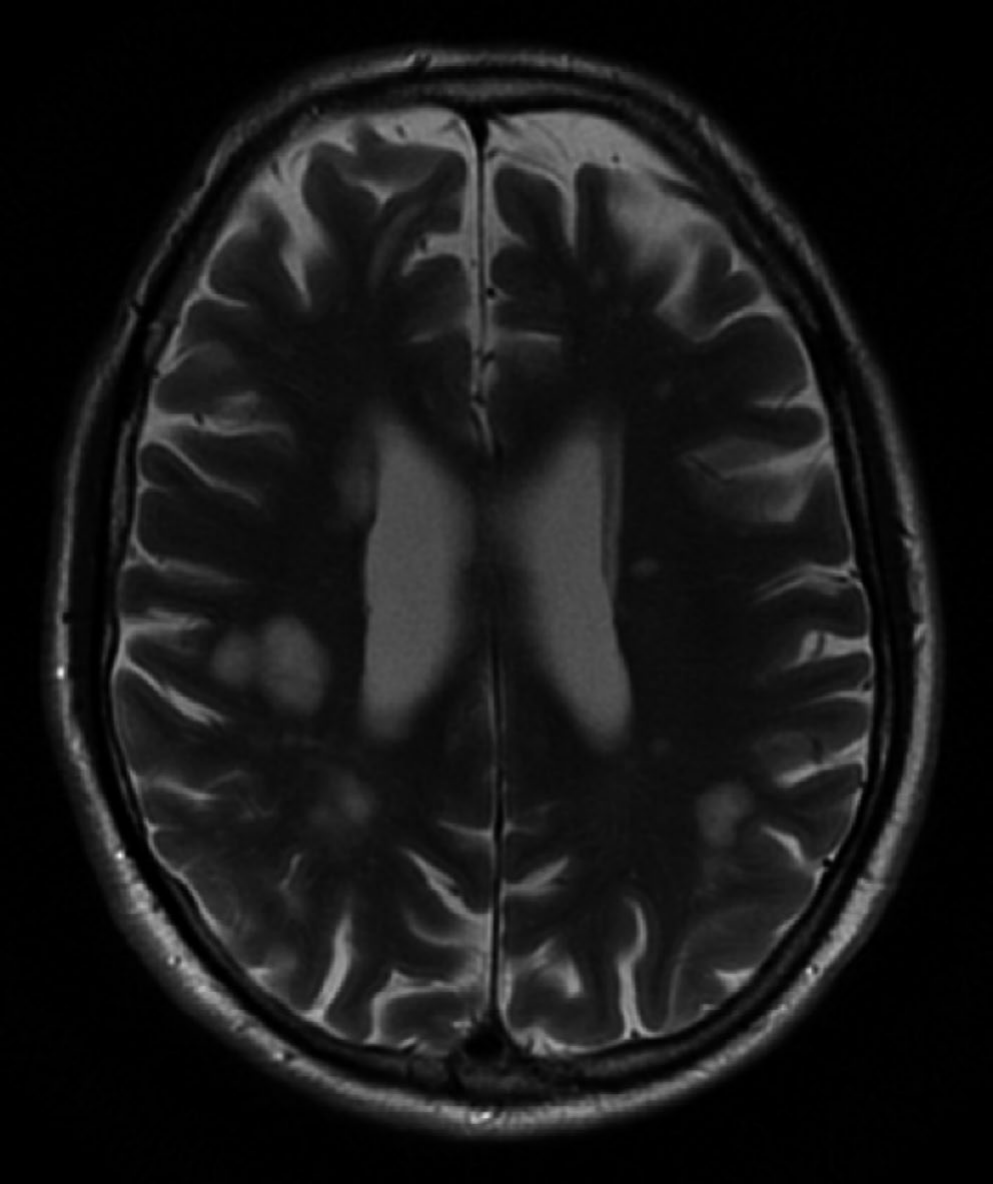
Magnetic resonance imaging of the brain showed numerous hyper‐intense T2 lesions within the subcortical white matter of both cerebral hemispheres
